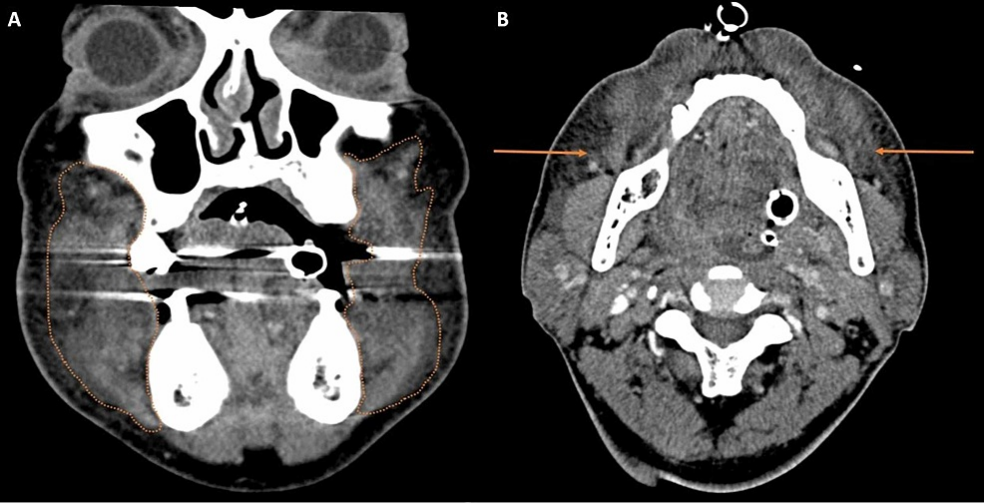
Coronal and axial enhanced CT A: Coronal enhanced CT demonstrating buccal mucosal swelling bilaterally representing angioedema (orange outline). B: Axial enhanced CT demonstrating findings of superficial and deep subcutaneous fat stranding representing subcutaneous angioedema (orange arrows). Evaluation of the tongue was limited due to beam hardening from dental amalgam and distortion from the endotracheal tube CT: computed tomography
